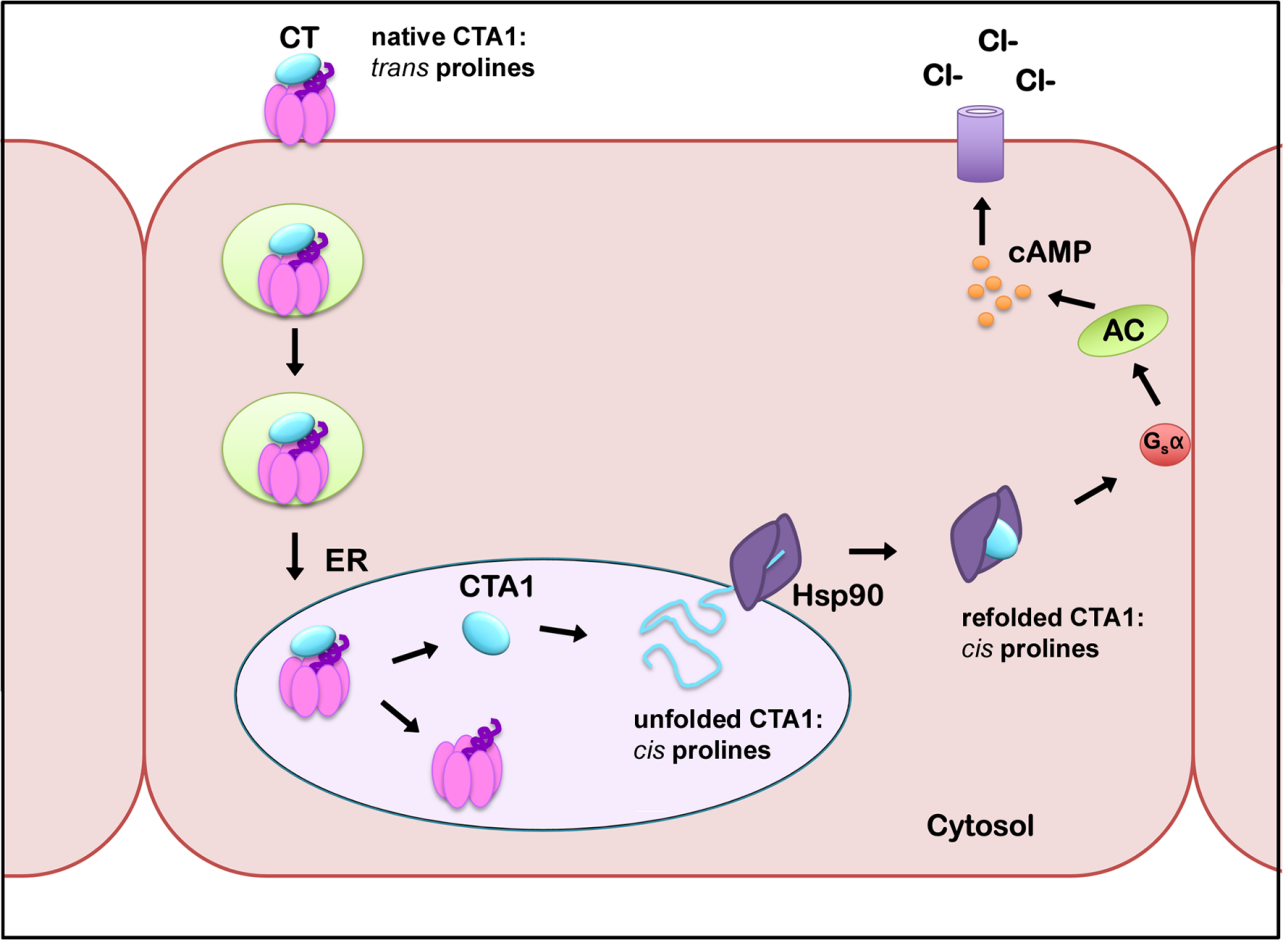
A model for the impact of proline *cis*/*trans* isomerization on CTA1-Hsp90 interactions. CTA1 enters the ER as a folded component of intact CT, with *trans* prolines in the RPPDEI motif. Separation of CTA1 from the rest of the toxin leads to its spontaneous unfolding and a hypothesized shift in its RPPDEI proline residues to the *cis* conformation. Those *cis* prolines are recognized by Hsp90 at the cytosolic face of the ER membrane after ERAD initiates the process of toxin translocation. Hsp90 then couples CTA1 refolding with CTA1 extraction from the ER translocon pore, but we predict refolding does not convert the RPPDEI prolines back to the *trans* state. Hsp90 thus remains in a stable complex with refolded CTA1 and its *cis* proline residues. Continued association of Hsp90 with CTA1 allows the otherwise unstable toxin to maintain its enzymatic activity in the host cytosol. AC, adenylate cyclase.
